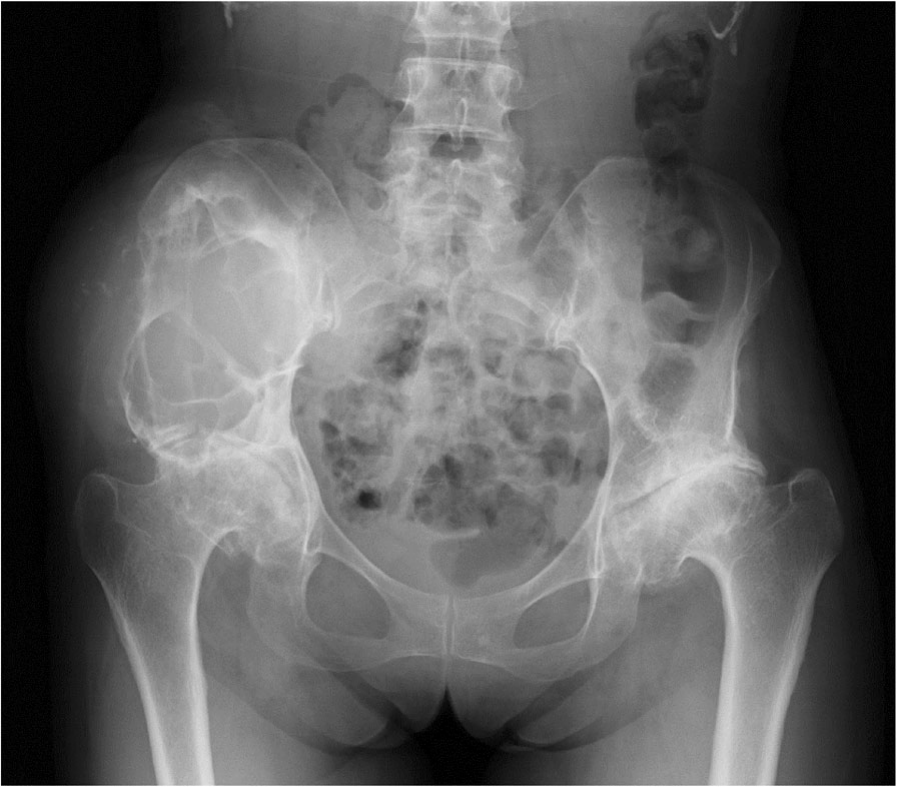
A plain radiograph of the ilium. Initial plain radiography showed marginal sclerosis and calcification inside the bone
